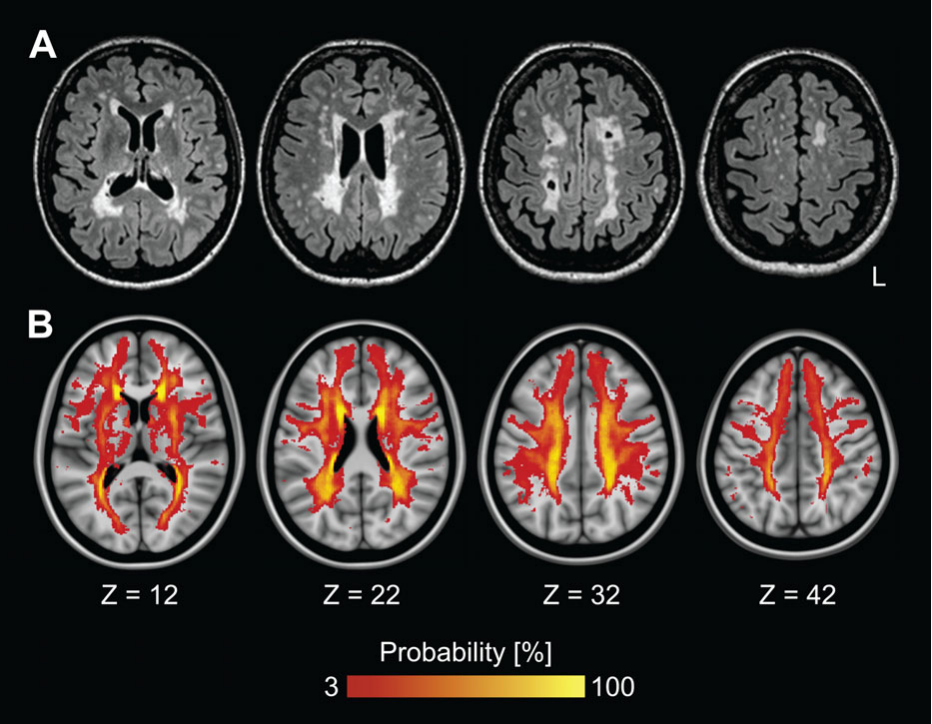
White matter hyperintensities in pure SVD. White matter hyperintensities on fluid‐attenuated inversion recovery (FLAIR) images. (A) Subject with median lesion load. (B) Lesion frequency map superimposed onto the Montreal Neurological Institute 152 standard brain template. L = left.
